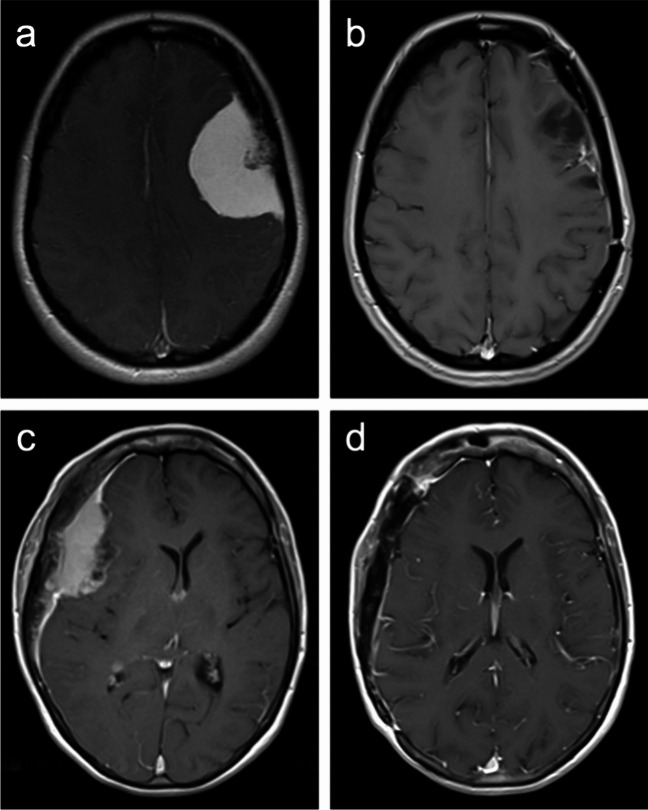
Case 4 (a&b): a, Preoperative T1-weighted MR image after contrast administration (T1 + c) in a 34-year-old woman with a CNS WHO grade 2 chordoid meningioma. There is evidence of tumour invasion into the calvaria. b, T1 + c MRI from the analogous location obtained 55 months post-operatively, demonstrating a section of the bone flap that had undergone EITC with no recurrence or bone resorption. Case 6 (c&d): c, Preoperative T1 + c MRI in a 63-year-old woman with an atypical meningioma. There is evidence of tumour invasion into the calvaria. d, T1 + c MRI from the analogous location obtained 48 months post-operatively, demonstrating a section of the bone flap that had undergone EITC with no recurrence or bone resorption
